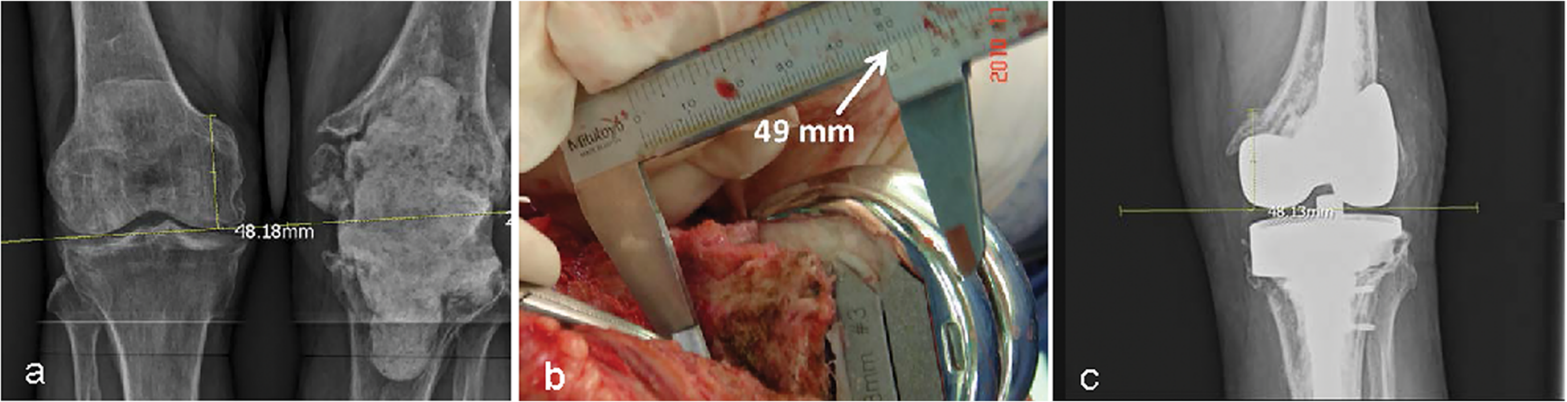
Application of the radiographic joint line distance (RJLD) measurement in revision knee arthroplasty, which is associated with significant bone loss. a The RJLD of the contralateral knee measured 48.18 mm on the scaled X-ray image. b During operation, an addition of a total of 16-mm thickness augmentation almost restored the planned joint line distance, as was shown by the intraoperative joint line distance measurement. c The RJLD (48.13 mm) measured on the postoperative radiograph confirmed the restoration of the joint line position in surgery
